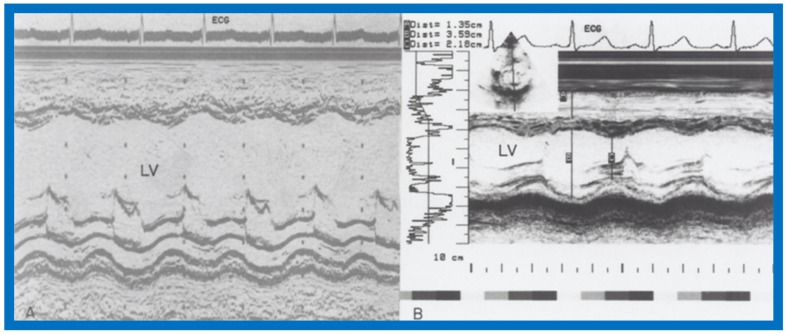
Selected M-mode recording from the parasternal short axis view of the left ventricle (LV) prior to (A) and following (B) hydrazine therapy. Note the significant improvement in the LV size and function.
